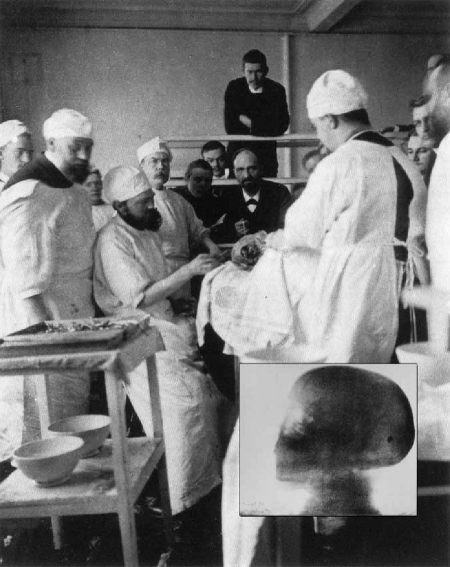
Professor Karl Gustav Lennander in 1897, removing a pistol bullet from the occipital lobe of the brain in a young man after the foreign body had been localized by X-ray. Photo: Medical Historical Museum, Uppsala.
